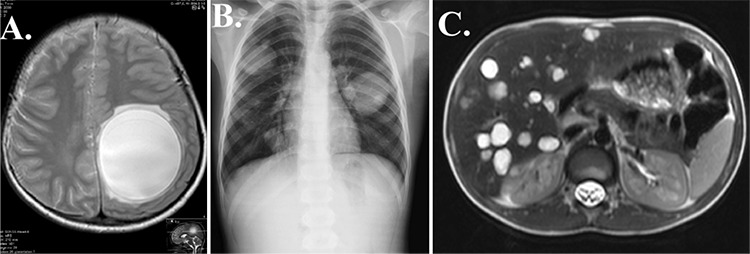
Images of the patient at presentation: Cranial magnetic resonance imaging T2-weighted axial image showing a large hydatid cyst with perilesional edema (a). Chest radiography showing multiple hydatid cysts (b). T2-weighted axial image at the level of the liver showing multiple hydatid cystic lesions (c).
